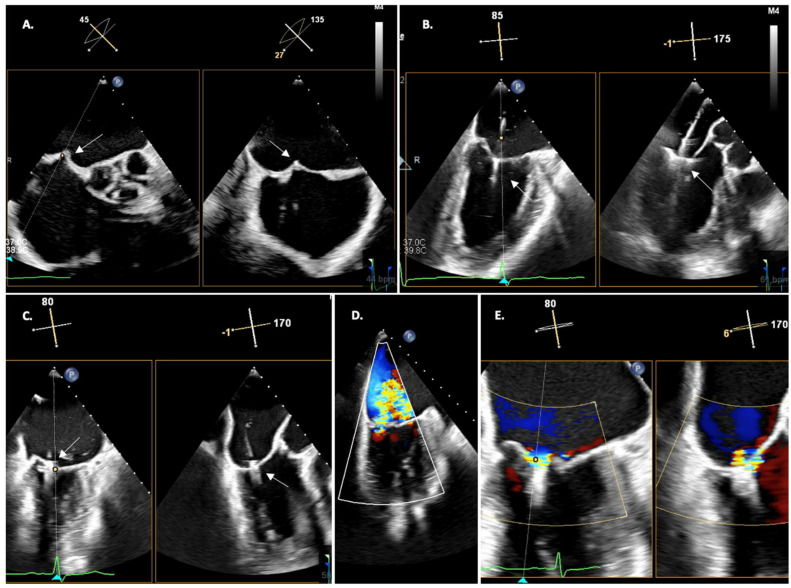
Echocardiographic procedural views of MitraClip implantation; (A) Trans-septal puncture (pre-puncture evaluation, white arrow); (B) Clip positioning (open clip pointed by white arrow); (C) Grasping (closed clip pointed by white arrow); (D) Pre-clip severe MR color flow; (E) Post-clip mild residual regurgitation.
